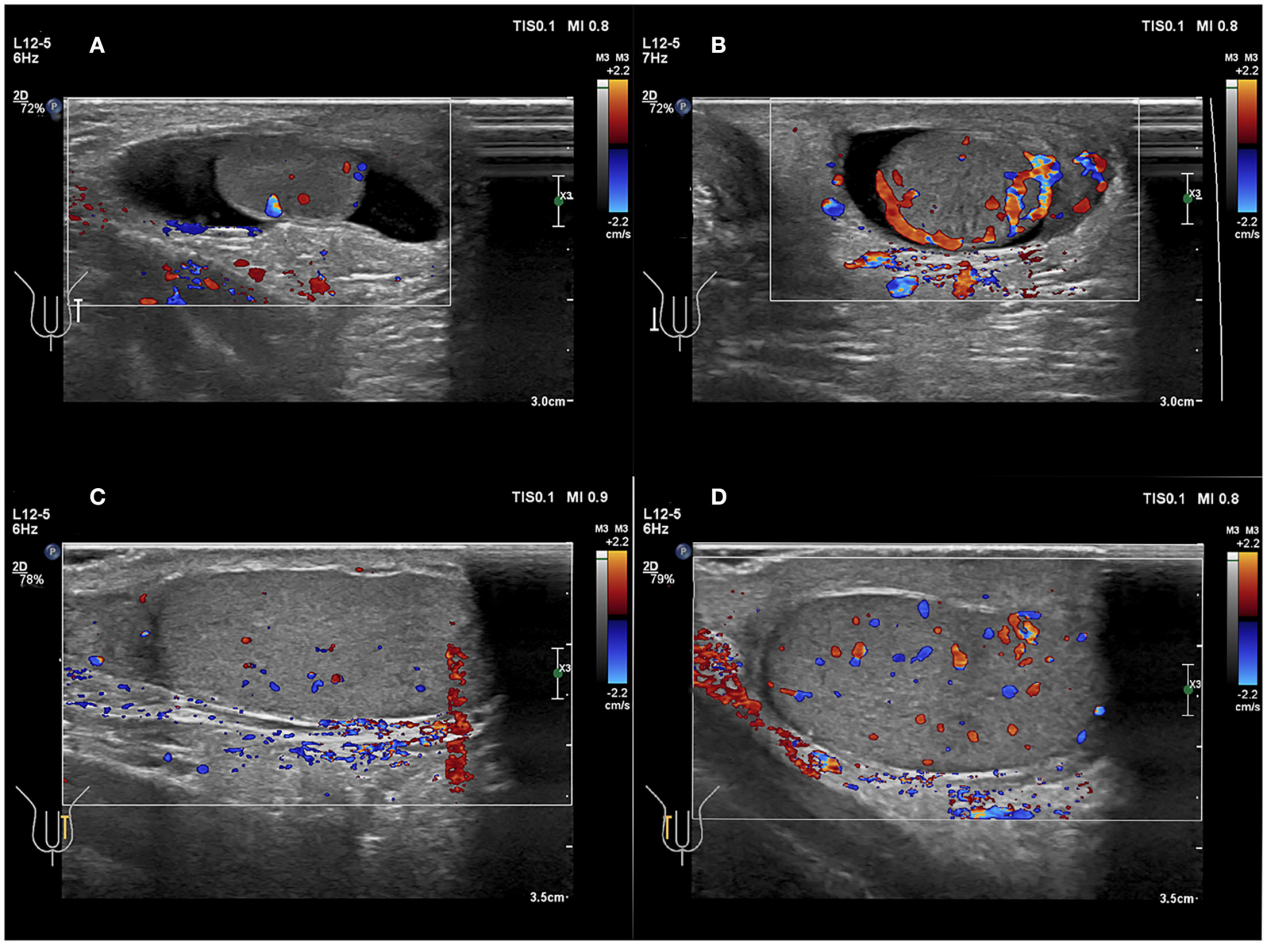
(A,B) A 49 days old boy with a first episode of acute right scrotal swelling for 4 days. (C,D) A 14 years 3-month-old boy with a first episode of acute right scrotal swelling for 7 h. (A,C) Normal testicle, Color Doppler showed blood flow of the testicle was normal. (B,D) Testicular torsion, testicular Color Doppler showed blood flow of the testicle was slightly increased.
